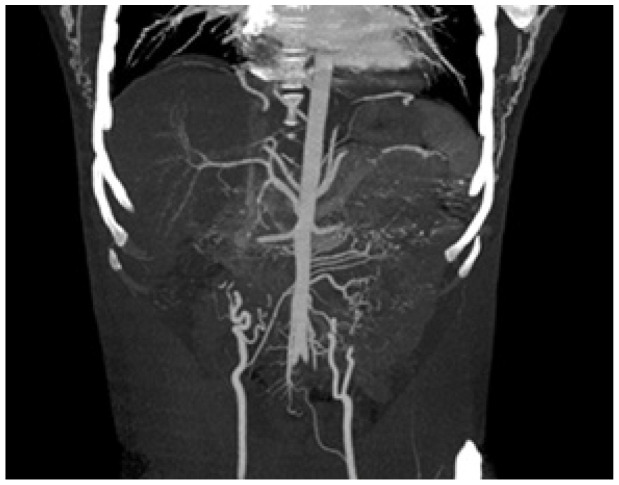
Computed tomography renal coronal section showing collaterals.
